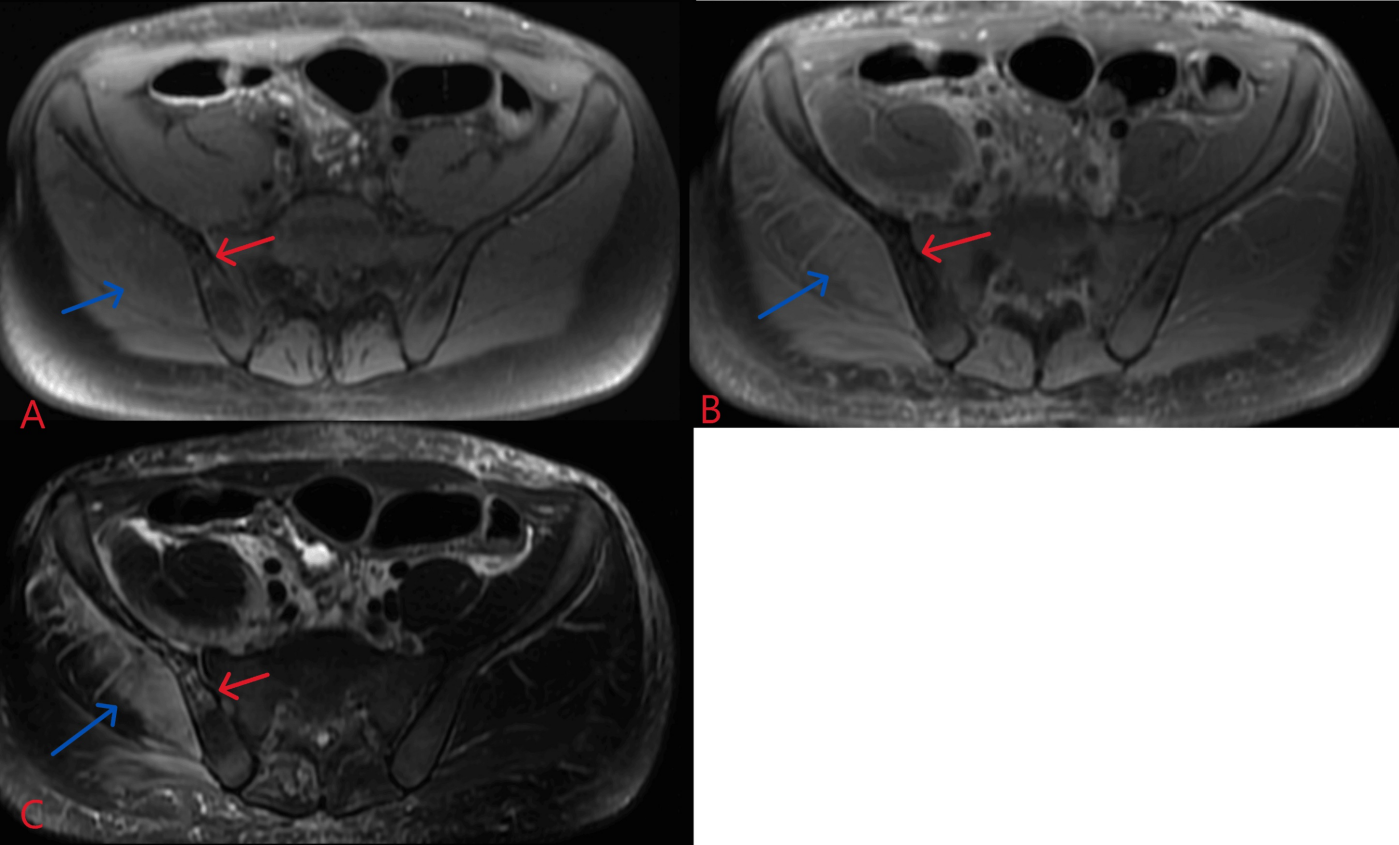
Initial MRI pelvis. T1 pre-contrast (A), T1 post-contrast (B), and T2 (C). There is a diffusely increased T2 signal in the muscles of the right hip (blue arrows), including the gluteal musculature, and the iliopsoas, which are enlarged and less well-defined relative to the contralateral musculature consistent with inflammatory changes/myositis. There is also an increased T2 signal of the subcutaneous tissues about the right hip. No discrete fluid collection is appreciated. Heterogeneous T2 hyperintense and T1 hypointense signal abnormality is visualized extending throughout the right iliac bone (red arrows). This corresponds to a region of decreased enhancement on the postcontrast sequence consistent with osteomyelitis with osteonecrosis.
